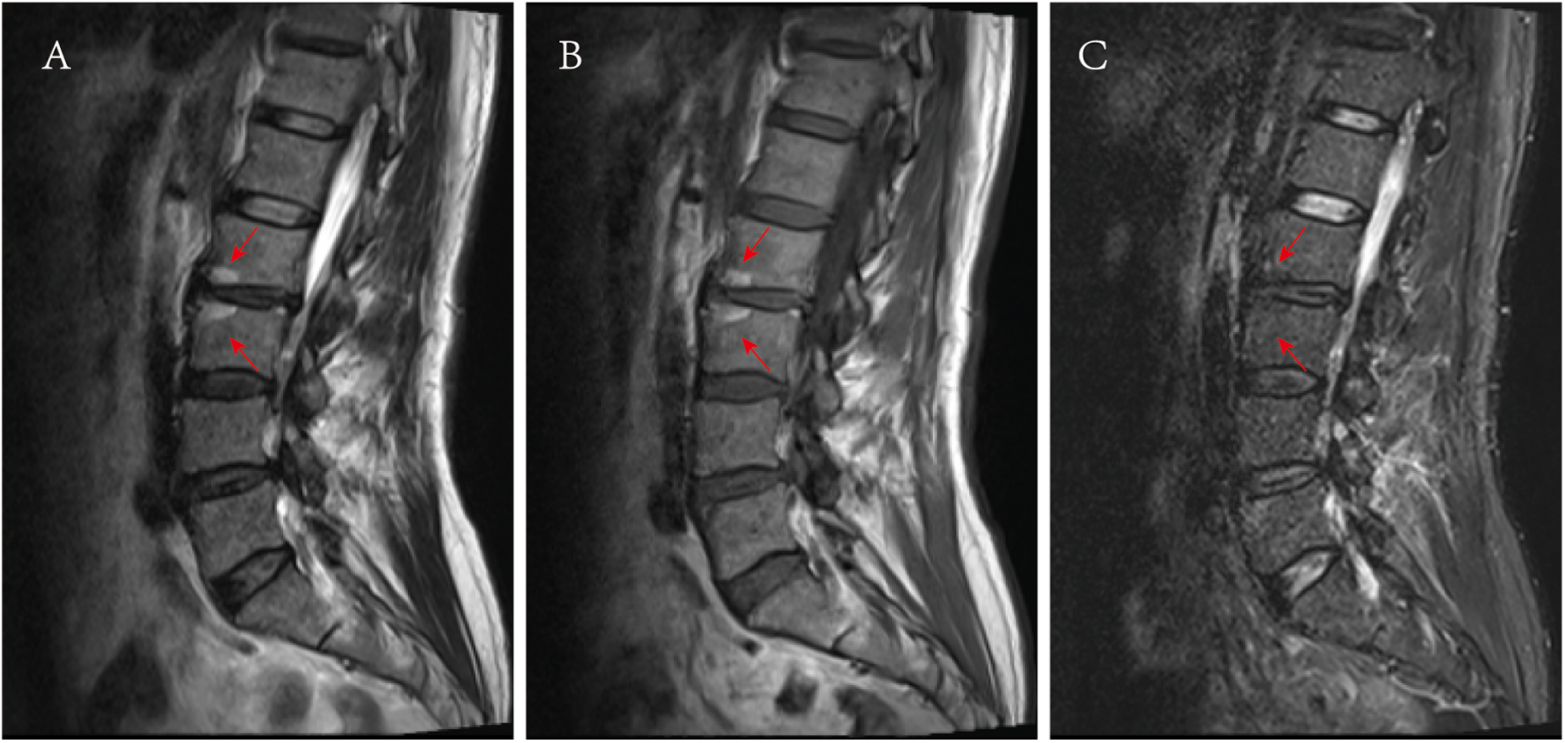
Images of Modic II. (A) Presented a high signal on the T2WI sagittal MRI, as shown by the red arrow. (B) Presented a high signal on the T1WI sagittal MRI, as shown by the red arrow, (C) A low signal was presented on the sagittal at fat suppression sequence MRI, as shown by the red arrow.
